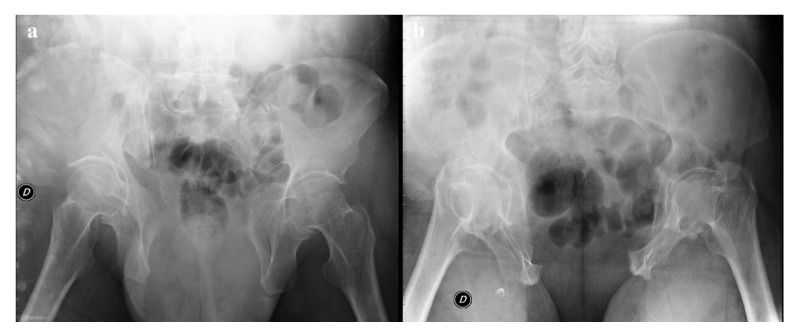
Plain radiography. The outlet view (a) and the inlet view (b) show a 5.2 cm diastasis of the pubic symphysis and anterior widening of the left-side sacroiliac joint. These findings have to be quickly reported to allow prompt treatment.
